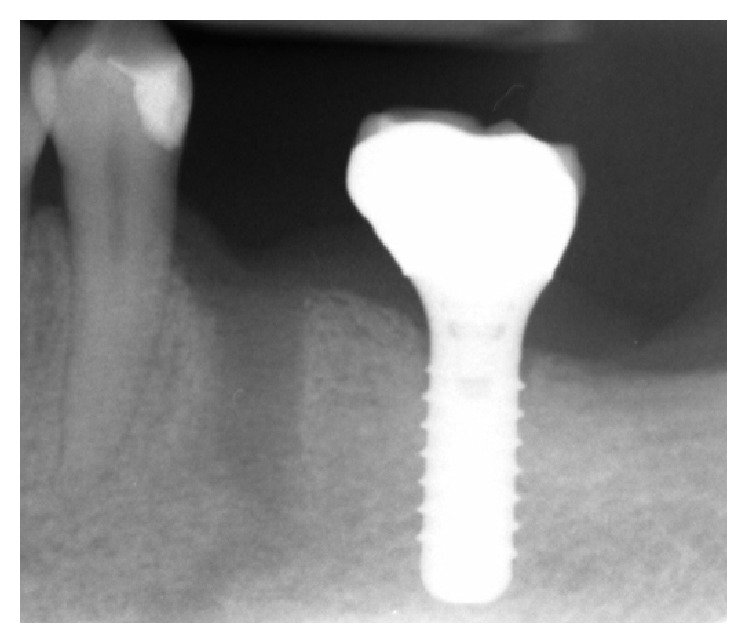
Radiographic exam after tooth extraction.
